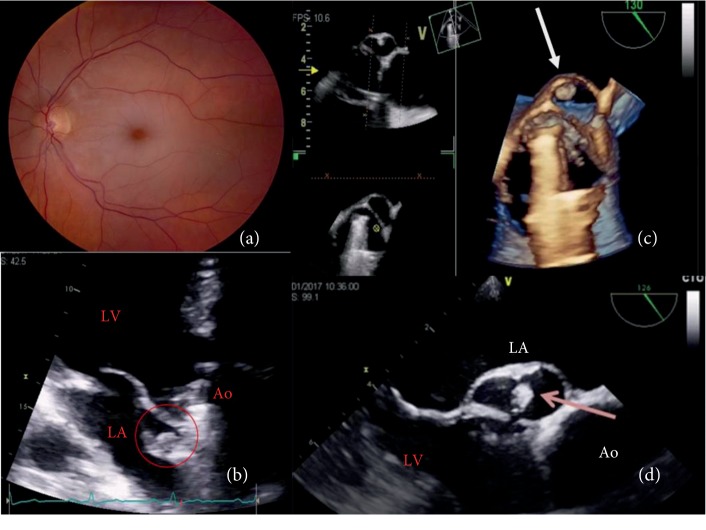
(a) Cherry red spot at the center of the fundoscopy. (b) Echolucent area in the mitral-aortic fibrosa with a hyperechogenic structure inside (red circle). (c) Reconstruction through transoesophageal echocardiography showing the mitral-aortic intervalvular fibrosa with a pseudoaneurysm (arrow) communicating with the left ventricle outflow tract and a free thrombus inside. (d) Transoesophageal echocardiography at 126° showing a free hyperechogenic highly mobile structure inside a pseudoaneurysm in the mitral-aortic intervalvular fibrosa, protruding into the LVOT through a small orifice. LA: left atrium; LV: left ventricle; Ao: ascending aorta.
